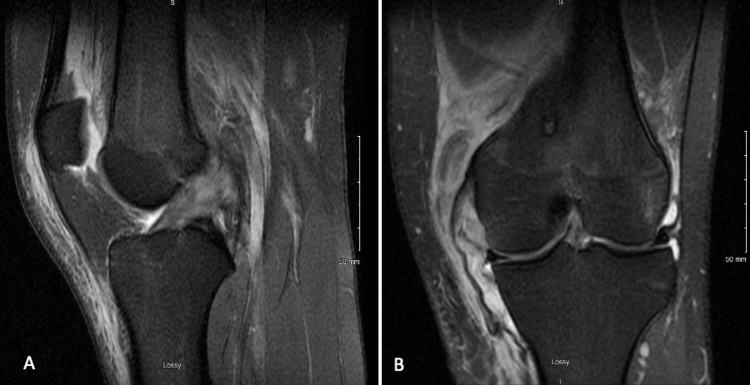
MRI demonstrating Grade III tibial-sided MCL tear (A) and an anteromedial ACL tear (B).
